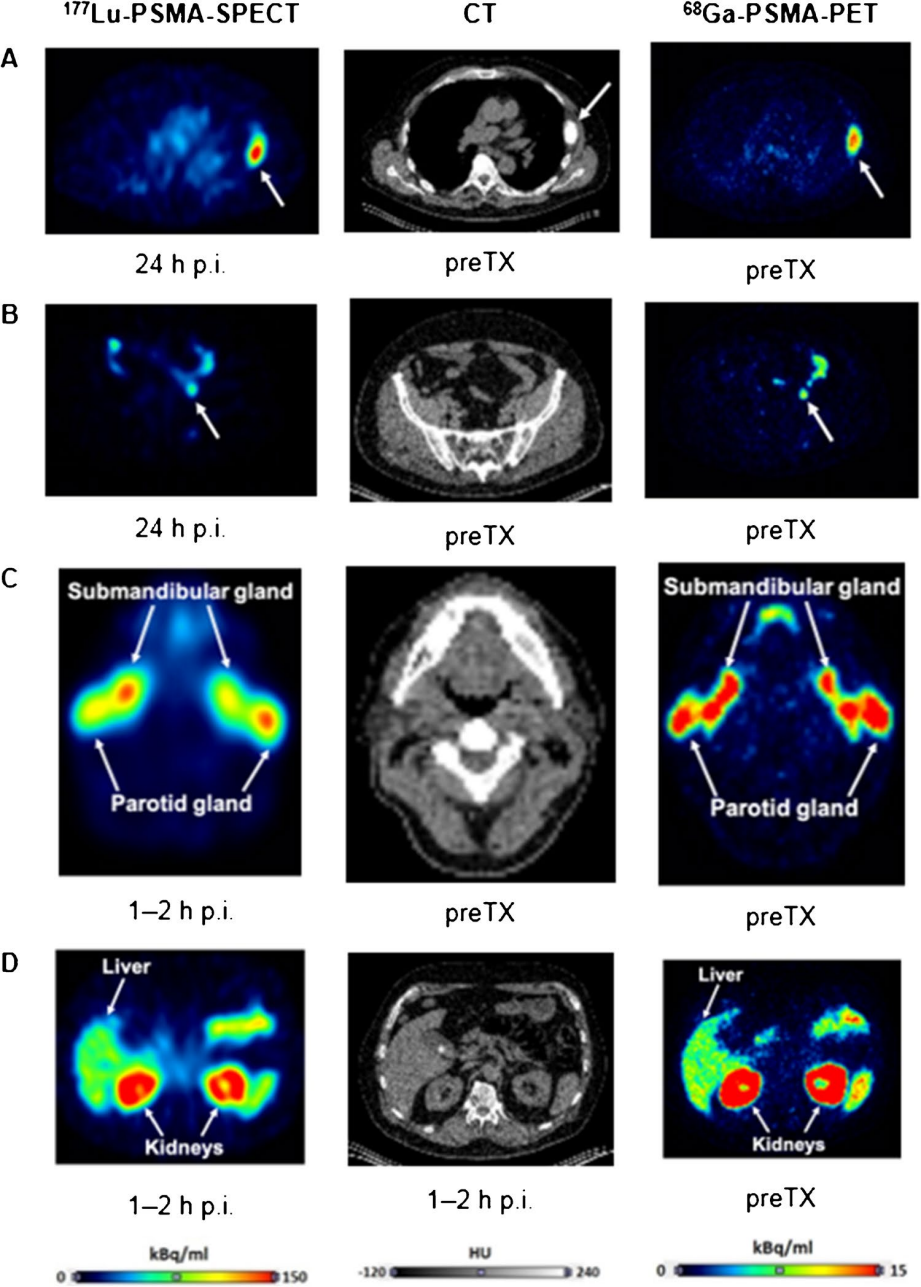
Representative images of [^177^Lu]Lu-PSMA-SPECT (left), CT (center), and [^68^Ga]Ga-PSMA-PET (right) for lesions and organs. A Bone lesion in abdomen region (visible on CT). B Lymph node lesion in pelvis region (not visible on CT). C salivary glands. D Kidneys and liver. The time points are chosen to visualize maximum uptake
